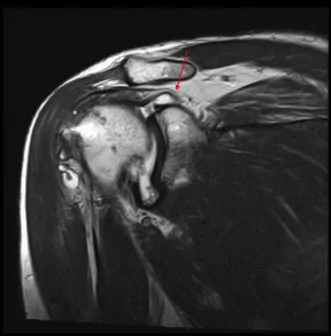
Postoperative magnetic resonance imaging at 1 year follow up shows adequate hamstring allograft integration. (arrow: glenoid border; *rotator cuff footprint).
